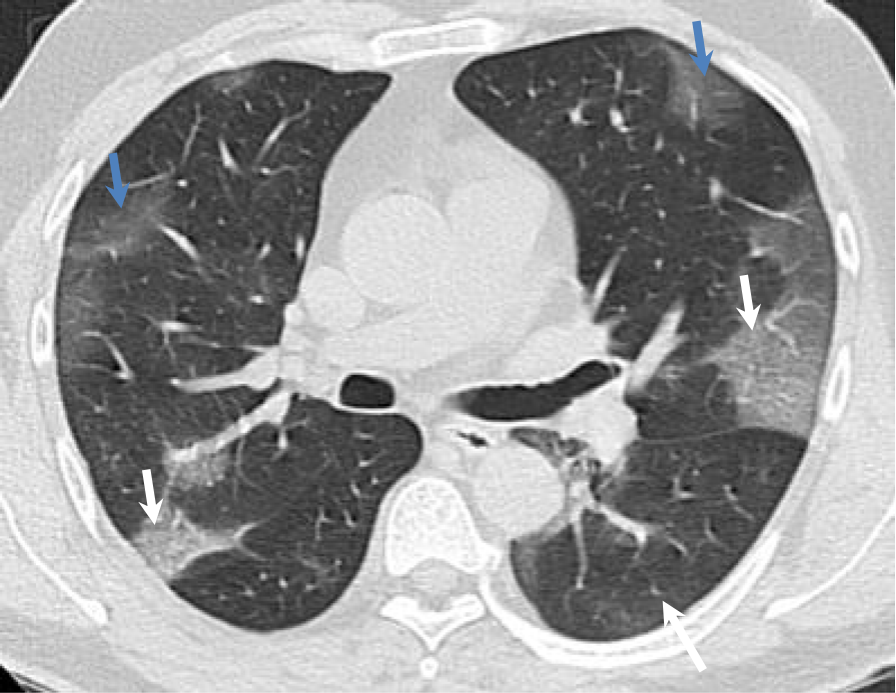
Image of a 49-year-old patient with a history of HIV in treatment with HAART, 6th day of illness with fever and dyspnea. The pulmonary parenchymal window thin section computed tomography shows the presence of ground glass opacities in the bilateral anterosuperior third (blue arrows) and the “crazy stone” pattern of bilateral posterior location (white arrows). There are no consolidations
